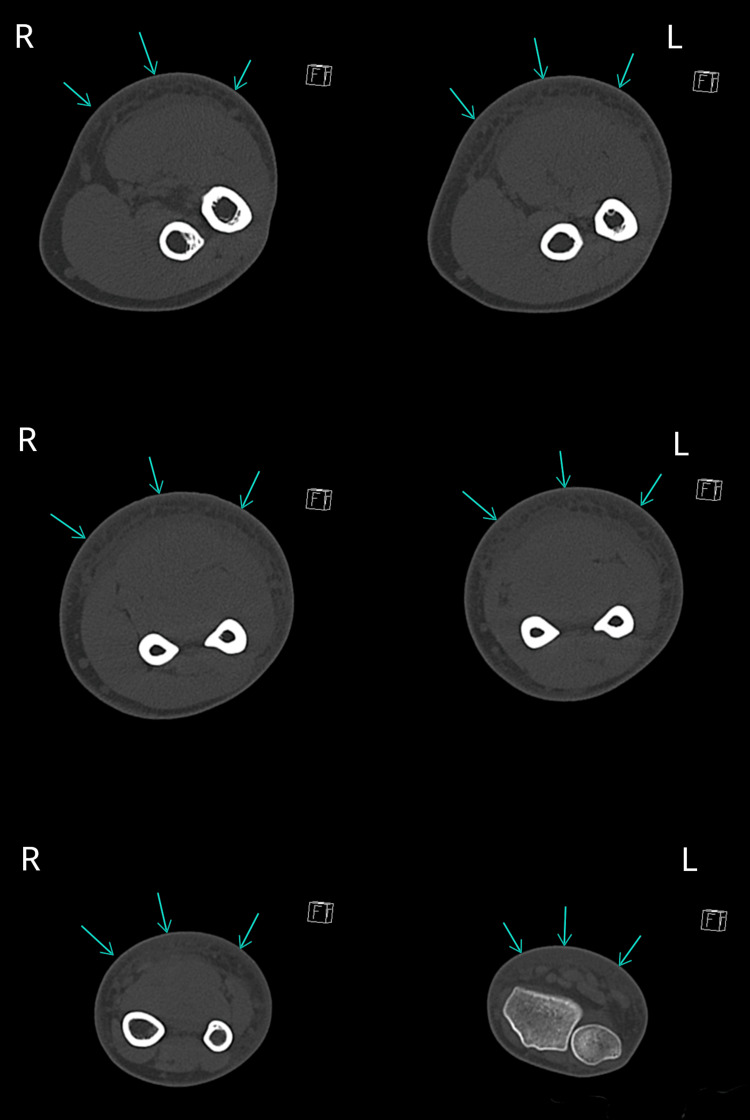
Axial CT of the forearm shows subcutaneous fat infiltration and collection along the muscle planes from elbow to wrist CT: computed tomography
